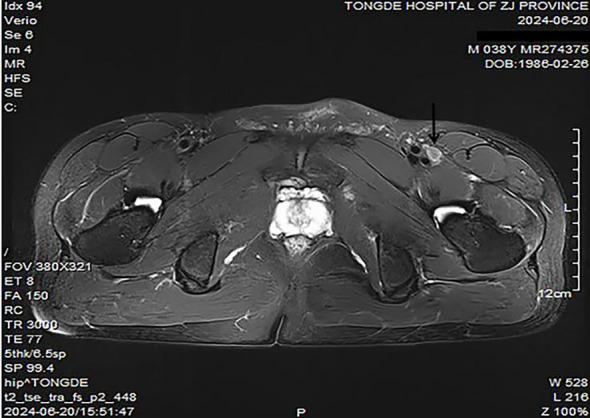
Contrast-enhanced MRI of the left femoral region. A fusiform lesion measuring approximately 3.0 × 2.0 × 1.0 cm is observed adjacent to the artery in the left inguinal region. The lesion shows an isointense signal on T1-weighted imaging and a slightly hyperintense signal on T2-weighted imaging, with well-defined margins. Following contrast administration, it demonstrates moderate enhancement with relatively weaker internal enhancement. The lesion is suggestive of a tumor of probable neural origin. The arrow indicates the lesion.
